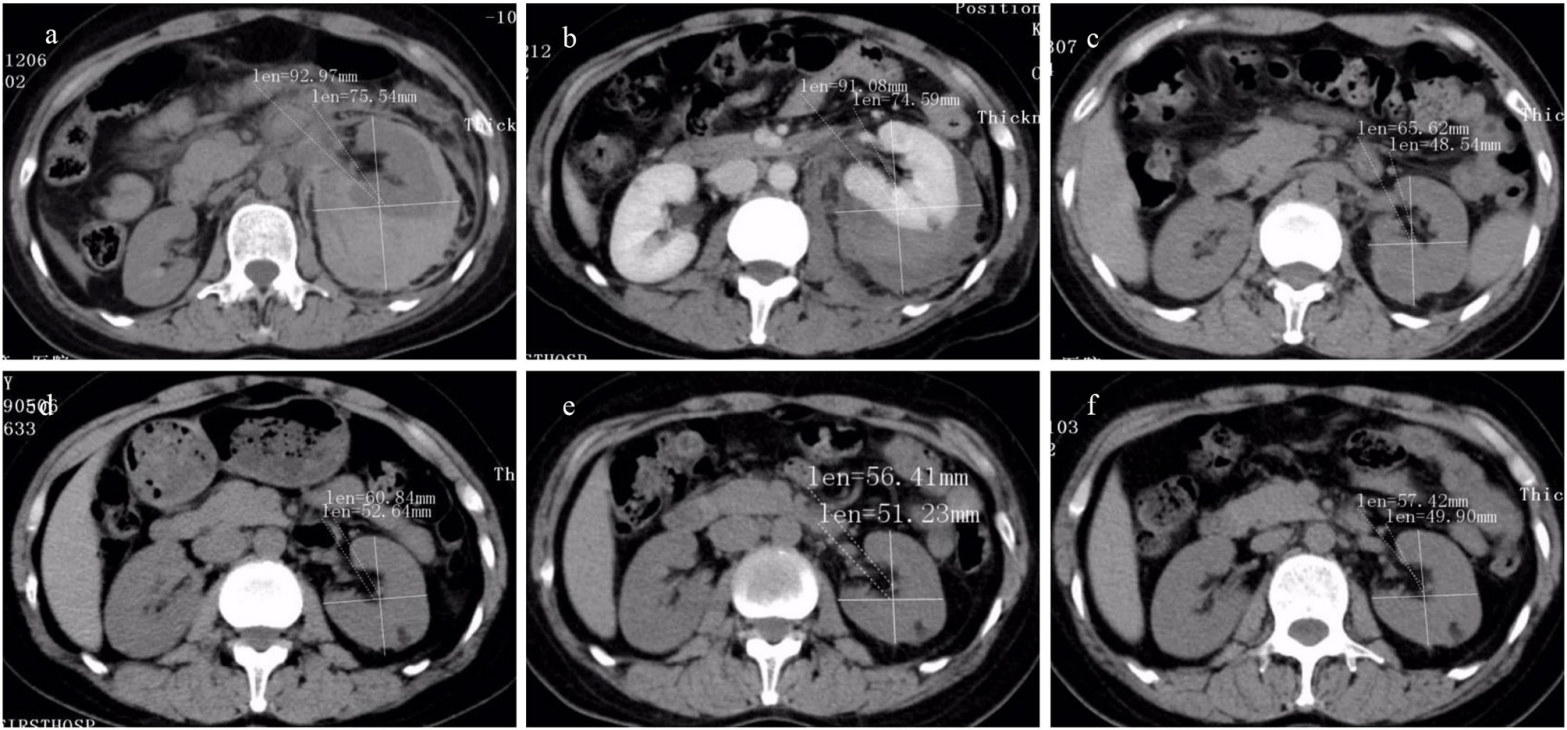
The CT findings of renal hemorrhage size owing to Wunderlich syndrome (WS) pre- and post-transcatheter arterial embolization (TAE) (the same patient as in Figure 2) were listed. High-attenuating hemorrhagic areas were seen around the kidney. (a–f) CT image obtained at pre-TAE, and at follow-up period of 1 week, 1 month, 3 months, 6 months, and 12 months. A significant reduction in hemorrhage size was observed.
